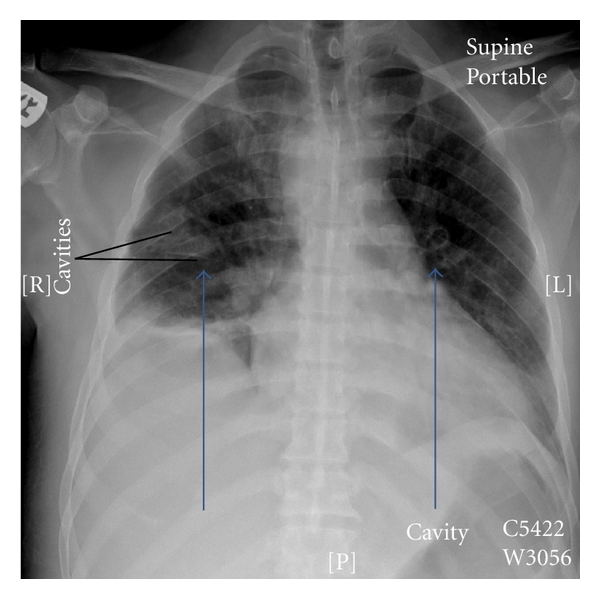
Chest X-ray of the chest showing multiple abscess cavities.
